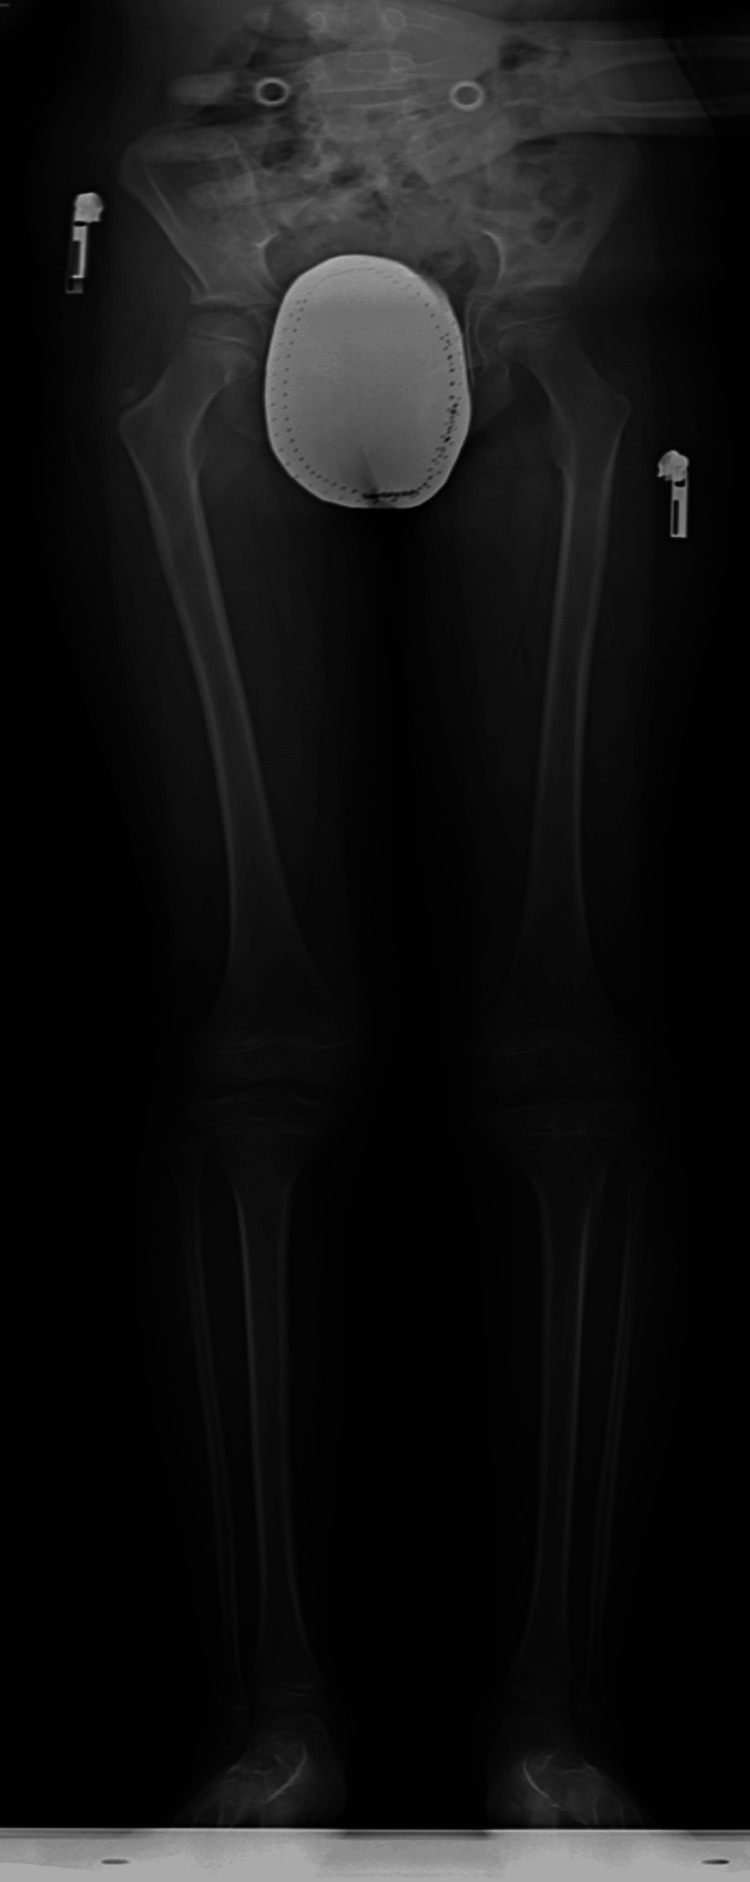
Final follow-up anteroposterior plain radiograph showing no lesion with no residual limb length deformity
